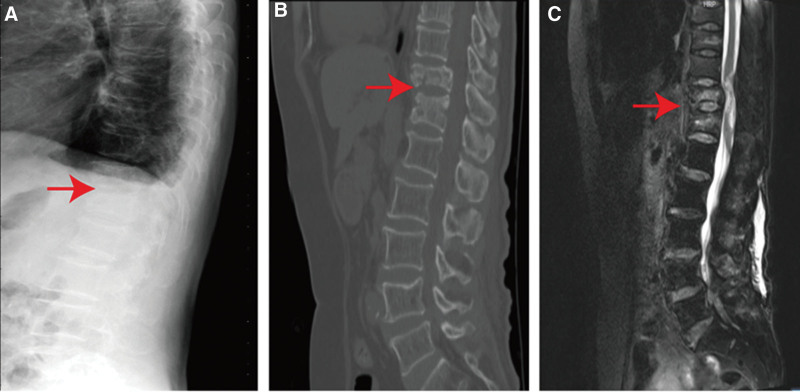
Radiographic examination of the patient with TSCI. (A) Lateral radiographs, the arrow points to the 11 to 12 thoracic fractures. (B) Computed tomography (CT) in the sagittal position, the arrow points to the 11 to 12 thoracic fractures. (C) Magnetic resonance imaging (MRI) in the sagittal position, the arrow points to injured thoracic spinal cord. TSCI = traumatic spinal cord injury.
